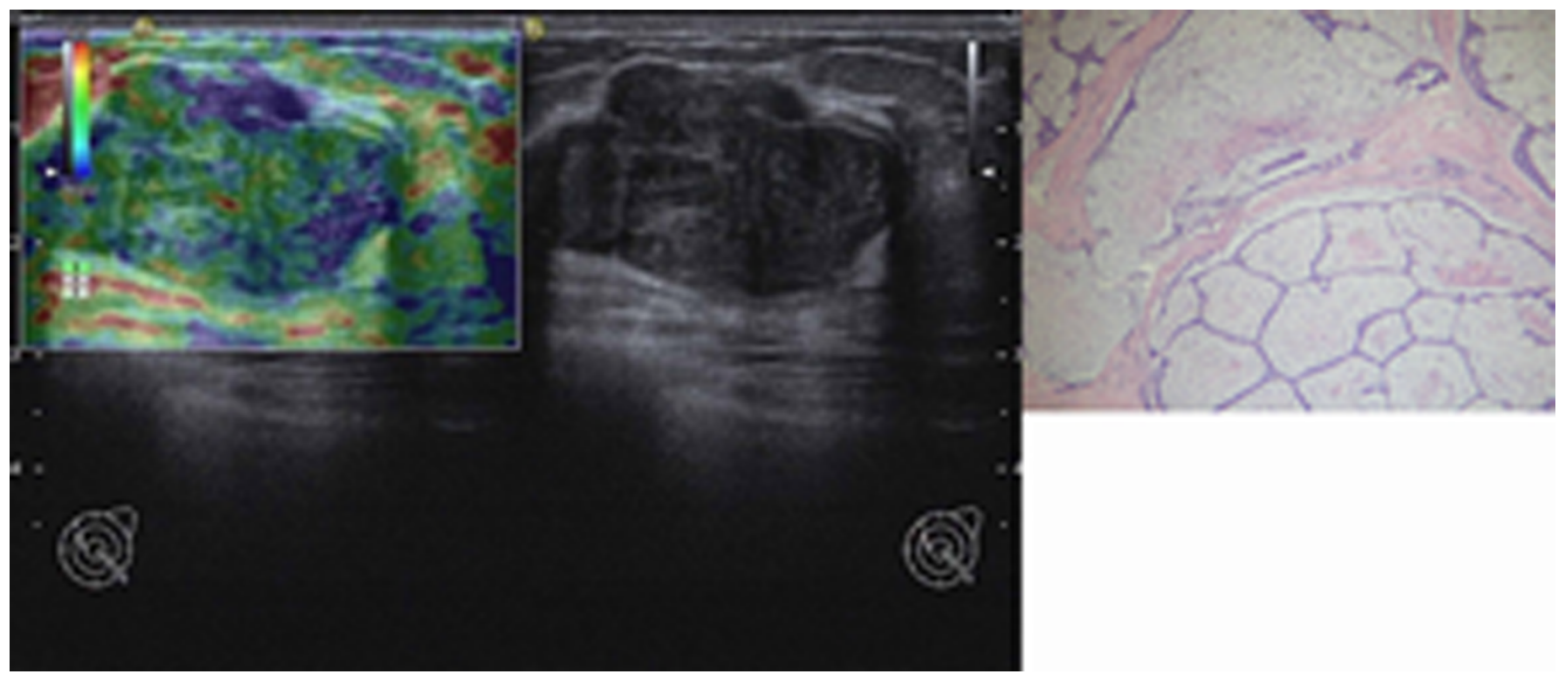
Image of a fibroadenoma in type I in a 25-year-old woman. Left: On elasticity image, most part of the lesion was green, but with some blue ultrasound elastography areas (score 2). Middle: Conventional B-mode image is shown. Right: Pathologic section of lesion is shown.
